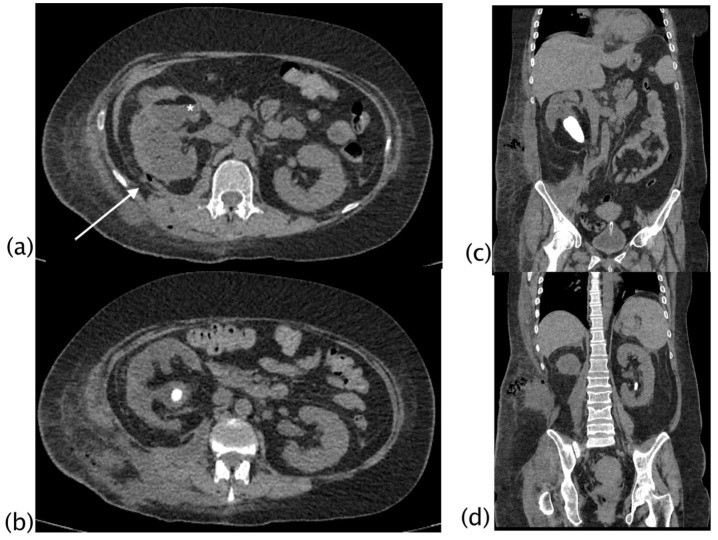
Xanthogranulomatous pyelonephritis Stage III—pararenal form. Non-enhanced CT examination: axial (a,b) and coronal MPR reconstruction (c,d). The right kidney is increased in volume with reduced parenchymal representation and a “contracted” appearance of the renal pelvis. The renal parenchyma is extremely thinned and characterized by the presence of multiple hypodense areas that cannot be better characterized without contrast agent. (a) Within the fluid collection at the anterior middle third of the kidney, a “layer” characterized by fluid (white asterisk) and adipose density (above white asterisk) can be noted, named “xanthoma” and characteristic of the disease. Staghorn lithiasis is present. No significant thickening of the perirenal fat tissue is noted. On the posterior profile of the kidney, a fistulous via with hydro-gas content is visible ((a), white arrow; (b)), which carries posteriorly into the psoas and dorsal muscles with fluid collection in the context of the muscle bundles. The fistulous via terminates in the subcutaneous flank soft tissues where fluid collection with gas is present ((d), MPR coronal).
